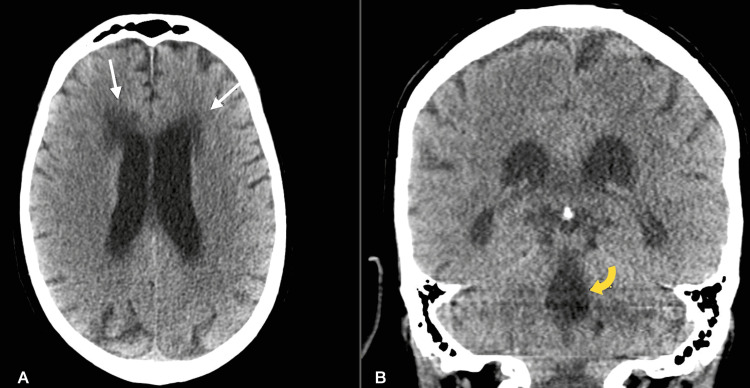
Axial CT image (A) shows new dilatation of ventricular system with white matter hypoattenuation adjacent to the ventricular system (straight white arrows), suggestive of acute hydrocephalus. Notice the dilated fourth ventricle (curved yellow arrow) on the coronal CT image (B), suggestive of communicating hydrocephalus.
